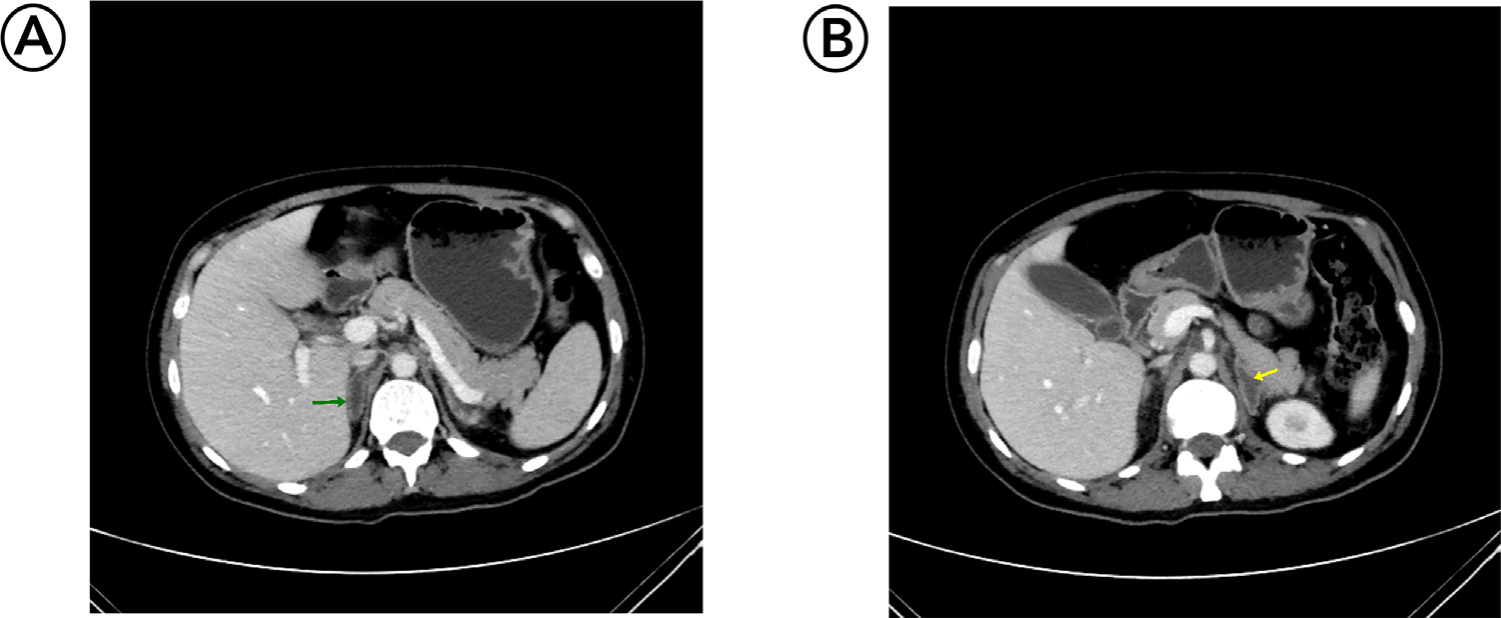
Axial sections of contrast-enhanced CT abdomen show central fluid density and peripheral enhancement of the right adrenal “green arrow” and left adrenal “yellow arrow,” indicating adrenal necrosis or hemorrhage.
